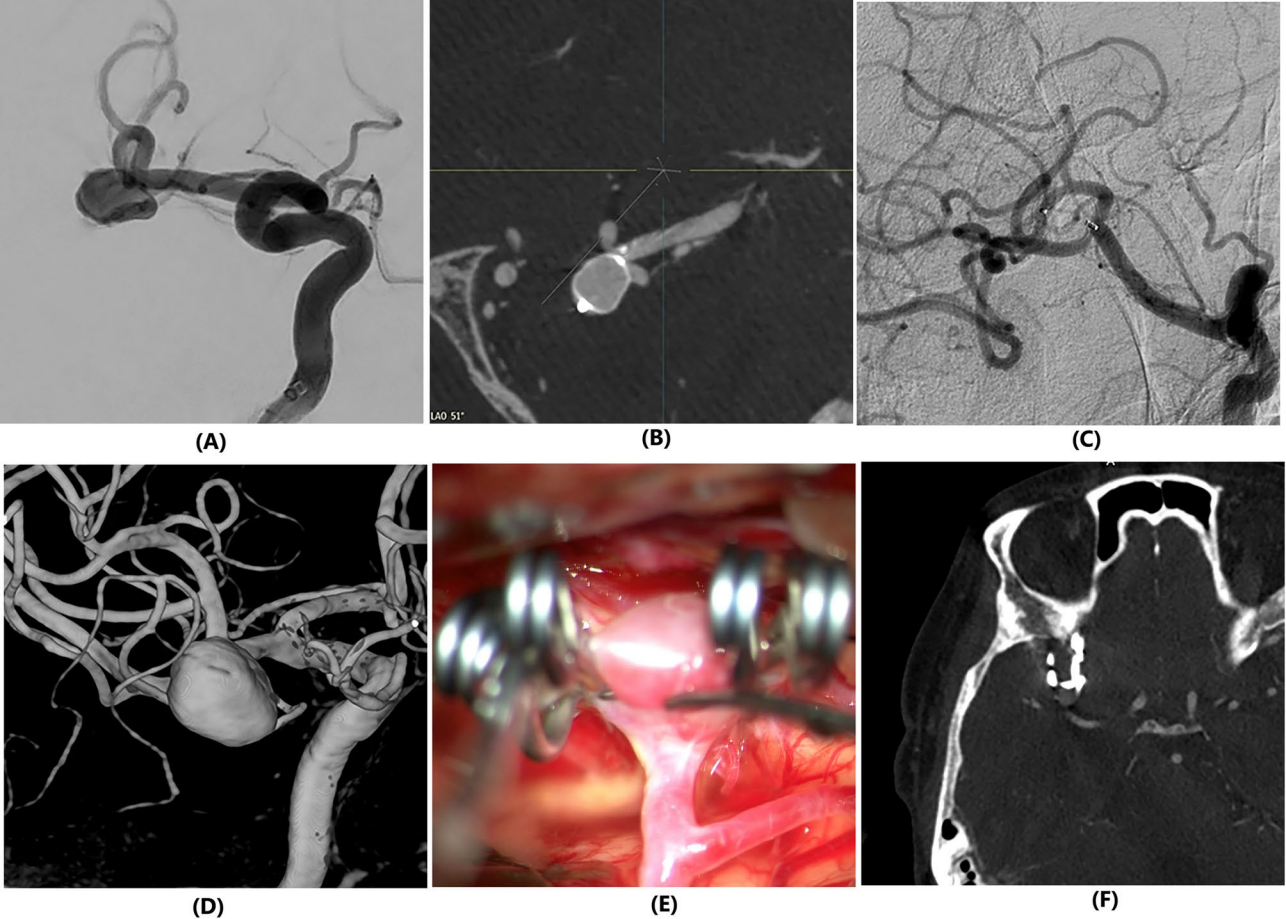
Case demonstration. Two-dimensional (2-D) digital subtraction angiography (DSA) showing a middle cerebral artery aneurysm planned for treatment with Woven EndoBridge (WEB) device (A). Intra-procedural flat-panel detector computed tomography image demonstrating placement of the WEB device (B). Twelve-month follow up 2-D DSA showing complete occlusion of aneurysm (C). Three-dimensional DSA showing a middle cerebral artery aneurysm planned for microsurgical clipping (D). Intraoperative view of aneurysm clipping (E). Twelve-month follow-up computed tomography angiography demonstrating a residual neck remnant (F)
